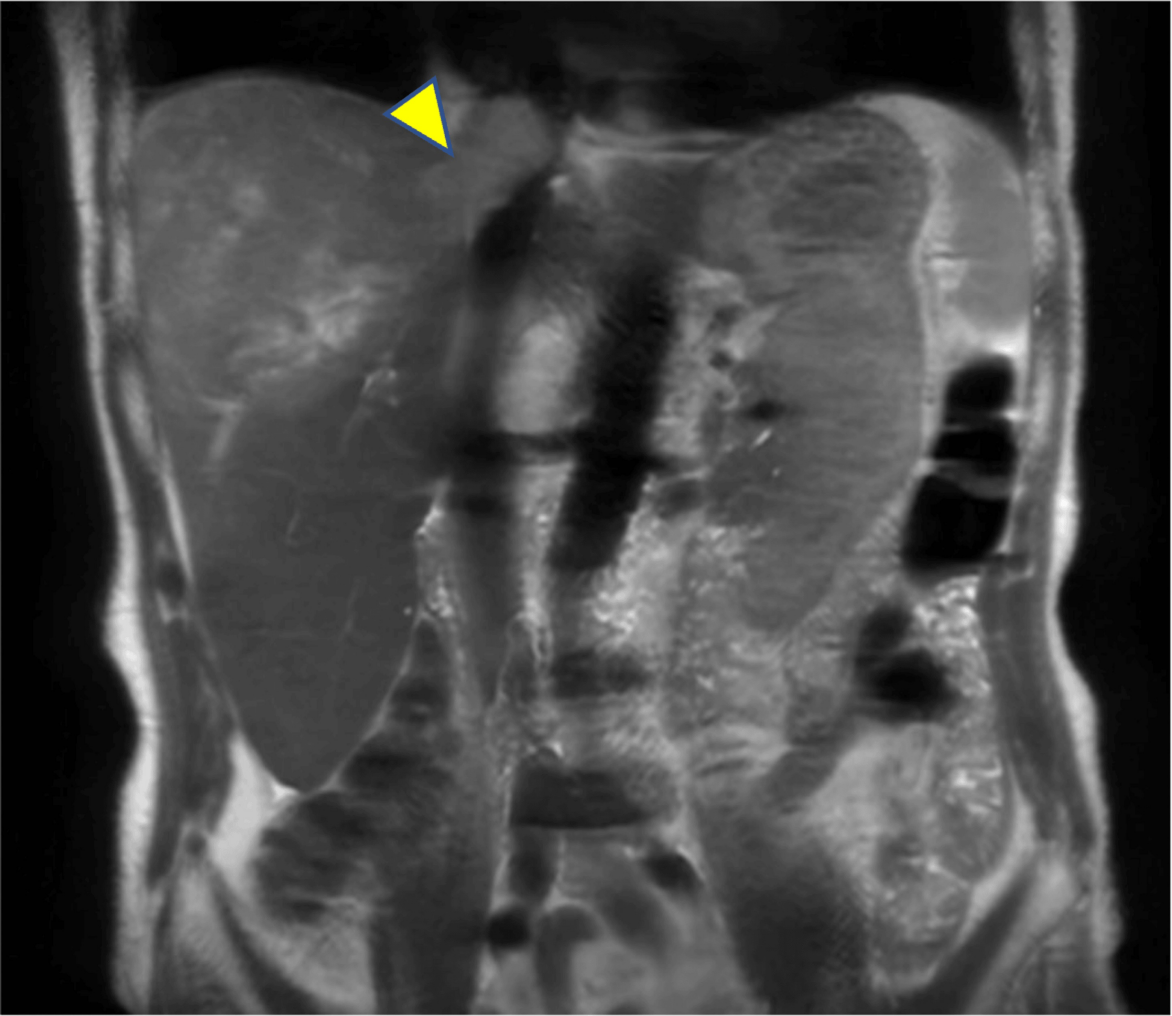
Contrast-enhanced magnetic resonance imaging (coronal plane) Coronal contrast-enhanced magnetic resonance imaging demonstrating an intraluminal tumor thrombus arising from the right hepatic vein and extending into the inferior vena cava (arrowhead), without radiological evidence of caval wall invasion.
